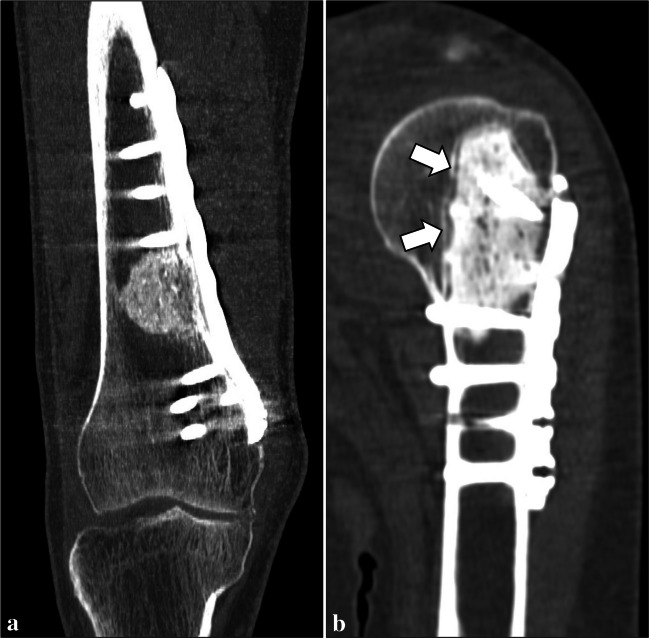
Normal imaging findings after curettage and bone grafting or cementation in a 73-year-old man (a) and in a 40-year-old woman (b), respectively. After curettage and bone grafting of an ACT of the distal femur, a coronal CT image shows normal graft integration into the host bone (a). After curettage and cementation of an ACT of the proximal humerus, a thin uniform radiolucent zone with sclerotic rim (arrows) around the cemented area is normal (b)
